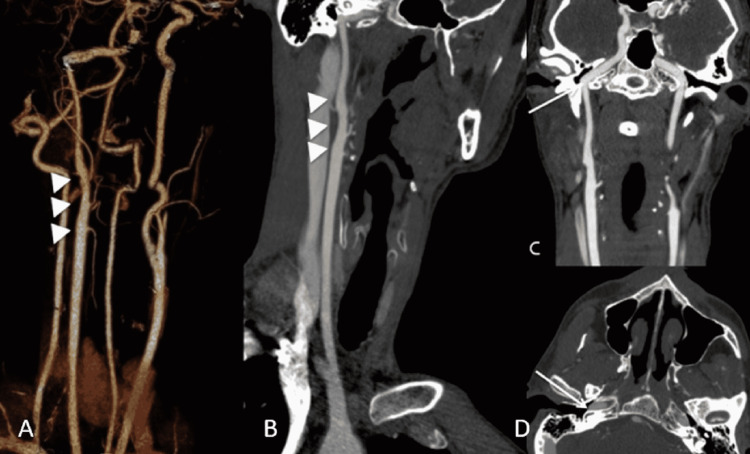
Oblique Volume rendered (A) and Maximum Intensity Projection (MIP) (B) images show the non-bifurcating carotid artery (NBCA) with external carotid artery branches (A, B, arrowheads. The inferior branch: Superior Thyroid Artery. Two middle branches with close origin: Lingual and Facial Arteries. The superior branch: Superior Temporal Artery). The Coronal MIP (C) and axial CTA (D) images further depict the lateralized course of the right internal carotid artery compared to the normal left side (arrows).
